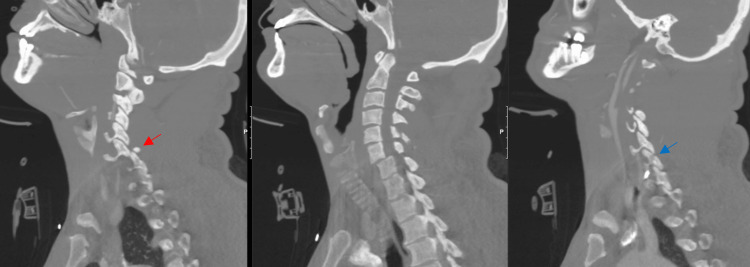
CT of the cervical spine with right parasagittal, midsagittal, and left parasagittal views of a cervical fracture/dislocation with a right C6-7 perched facet (left image, red arrow) and left C6-7 lateral mass fracture (right image, blue arrow).
